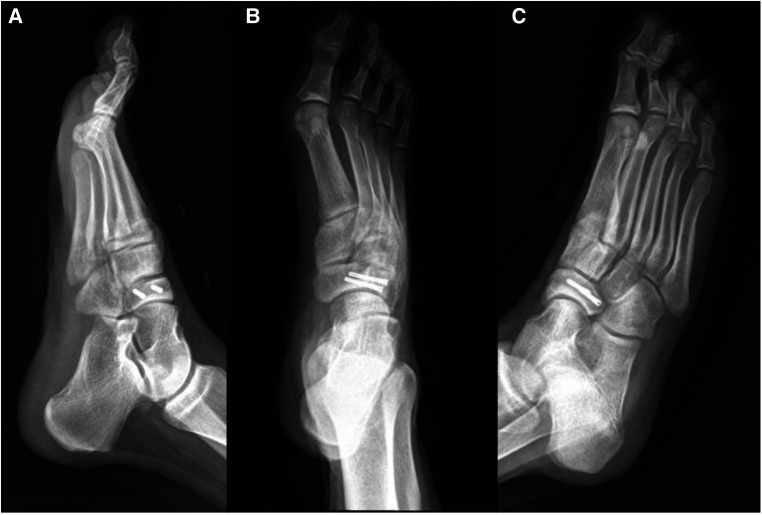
Immediate postoperative imaging data. (A) Non-loaded lateral x-ray of the right foot; (B) anteroposterior x-ray of the right foot; (C) oblique x-ray of the right foot.
